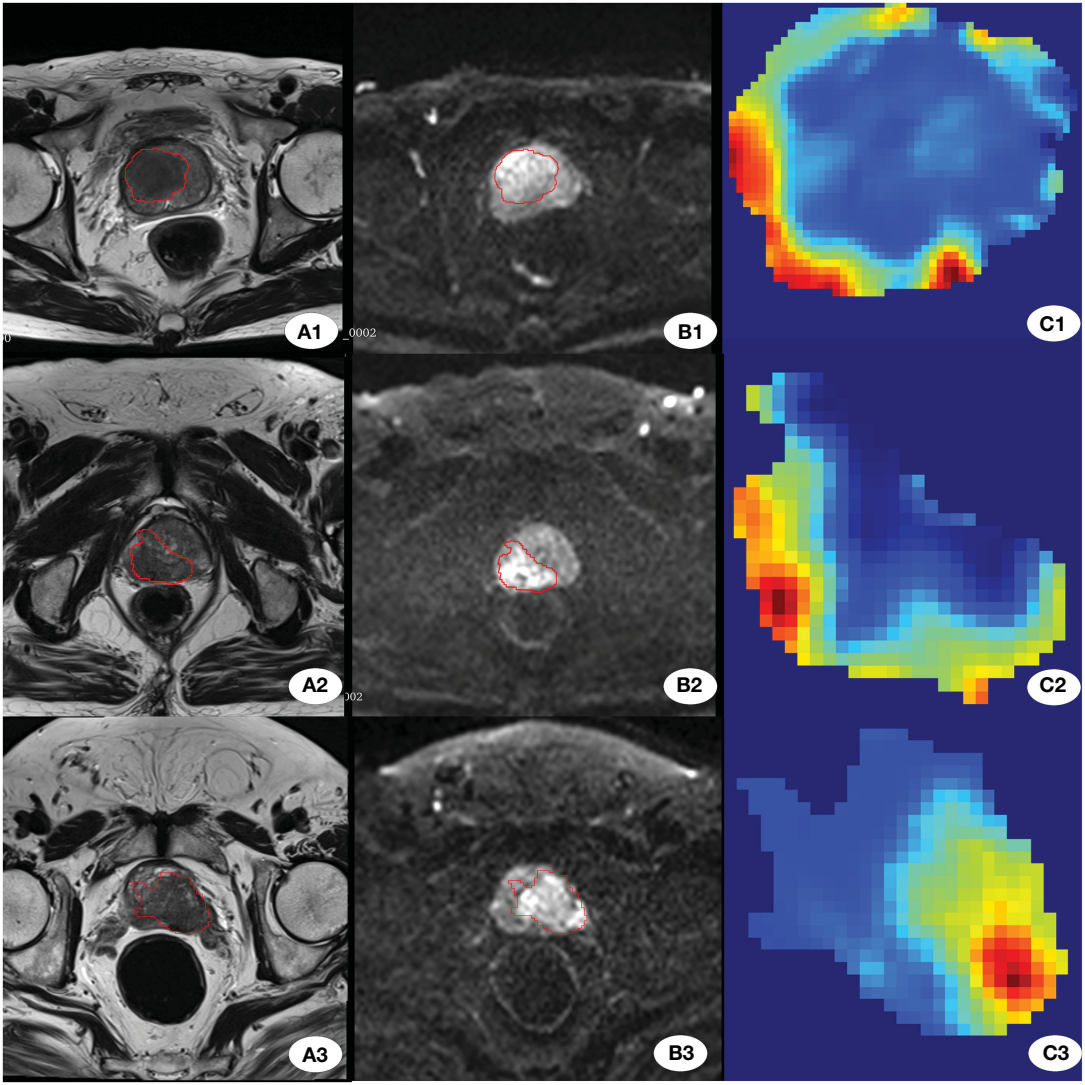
Grad_GAM. (A1-A3) show T2-weighted images from three different patients with BM. (B1-B3) display the corresponding DWI images from the same three patients. (C1-C3) present the Grad-GAM images of the largest cross-sectional area of the tumor for each patient.
